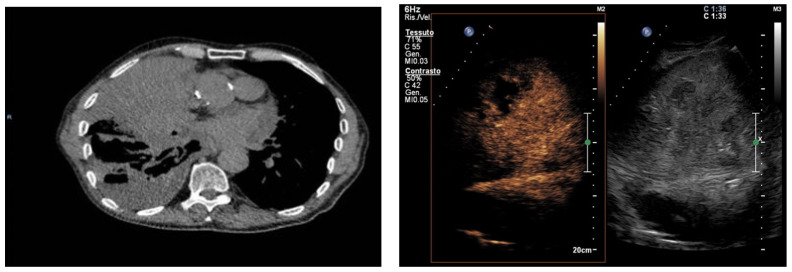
Multimodal imaging of extensive pulmonary consolidation with hepatization and central necrosis. Axial computed tomography (CT) image showing a large pulmonary consolidation with a hepatized appearance. Contrast-enhanced ultrasound (left) and corresponding B-mode ultrasound (right) demonstrating a solid-appearing consolidation with a non-enhancing intralesional area consistent with necrosis.
